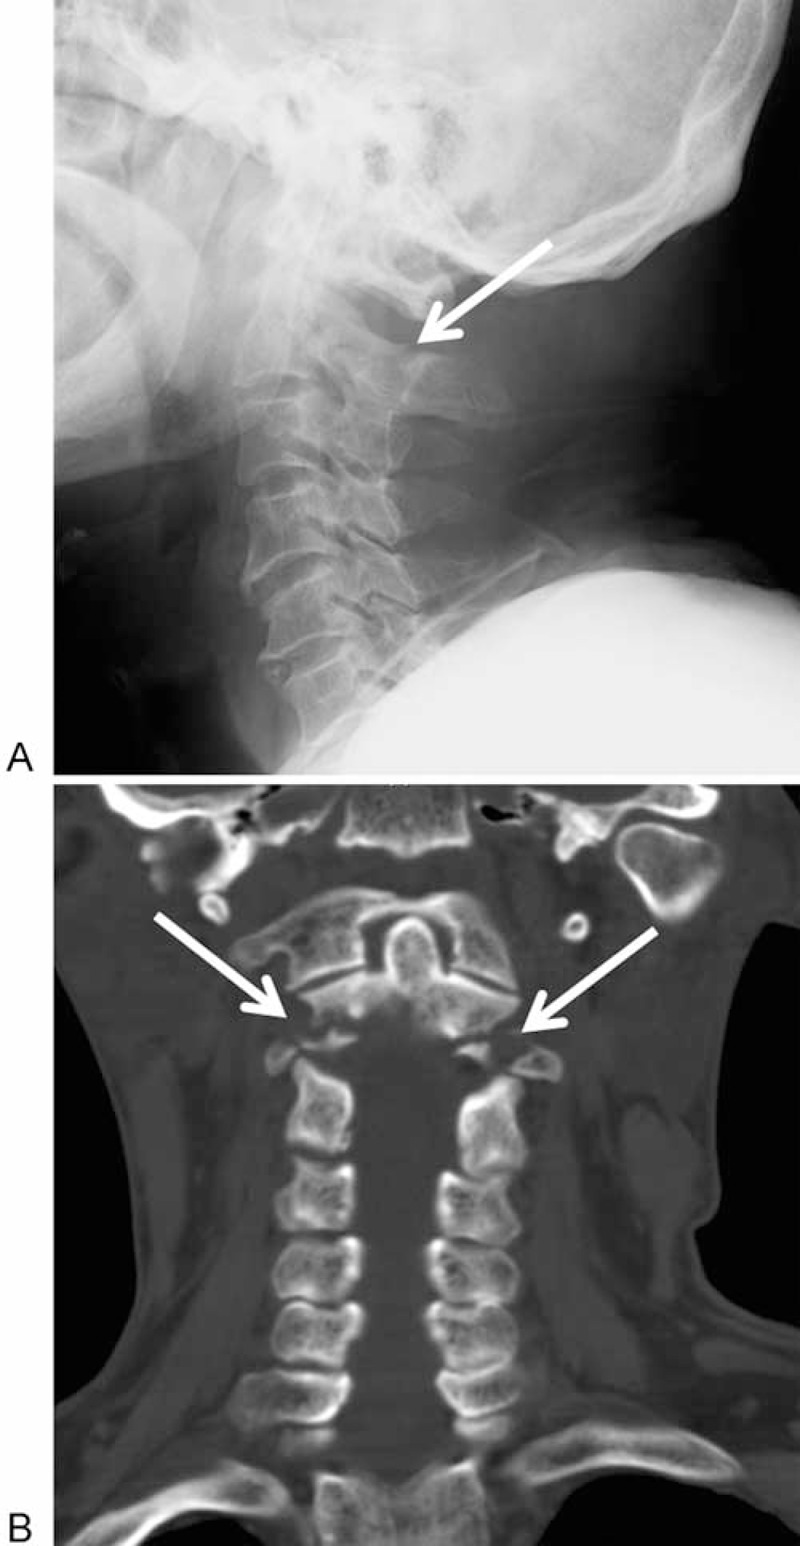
Plain radiograph and CT of the cervical spine in a 67-year-old male with cervical fracture (C2–C4). He fell down stairs while intoxicated. Two days later, he visited our ED with a chief complaint of neck pain. (A) Lateral plain radiograph of the cervical spine shows a fracture through the lamina of the atlas (arrow). (B) Coronal CT shows fractures through both sides of the laminae and arch of the atlas (arrow).
